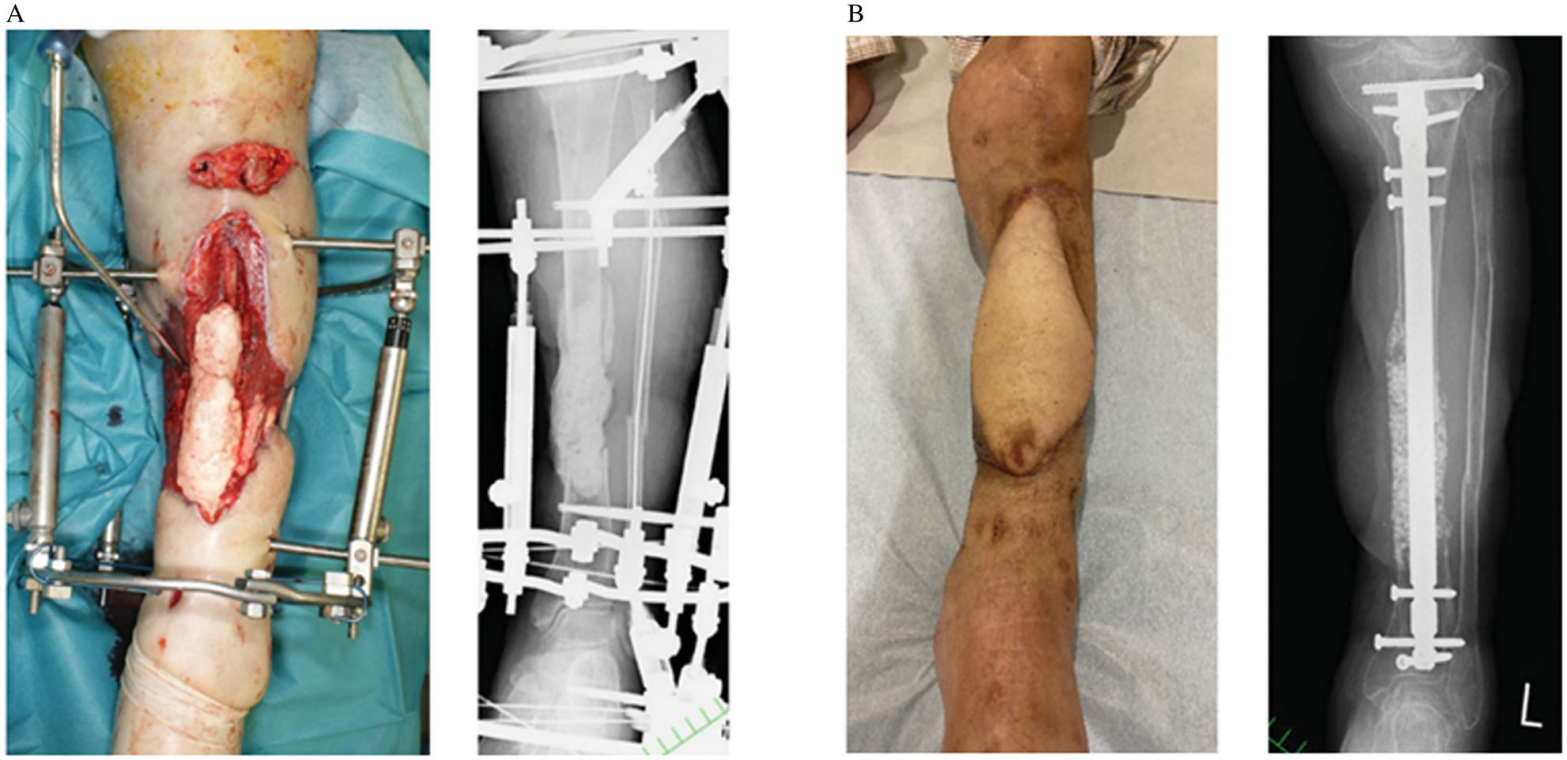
Case 2: (A) Preoperative clinical and radiographic findings. (B) Postoperative findings at six months.
